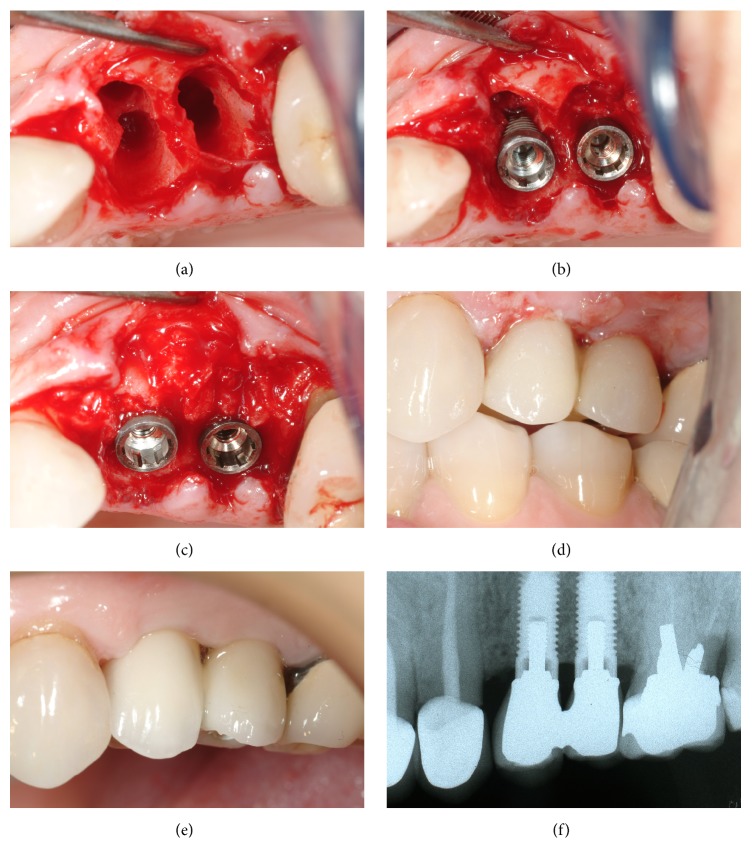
(a) Two adjacent extraction sockets in maxilla. (b) One bimodal and one proactive implant installed into fresh extraction sockets. (c) The bone defects were treated with autologous bone particles. (d) Temporary prosthesis without occlusal contacts. (e) Final restoration. (f) The radiograph after 3 years.
